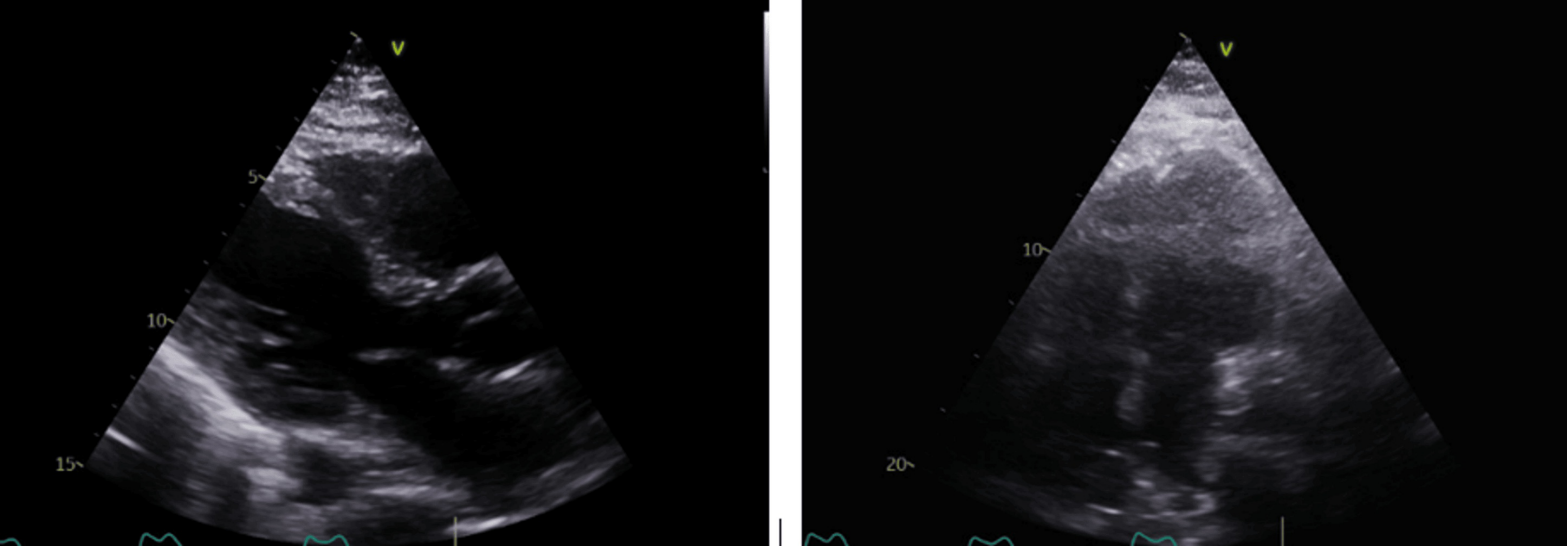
Transthoracic echocardiogram (showing mildly reduced left ventricular systolic function (left ventricular ejection fraction = 45%).
